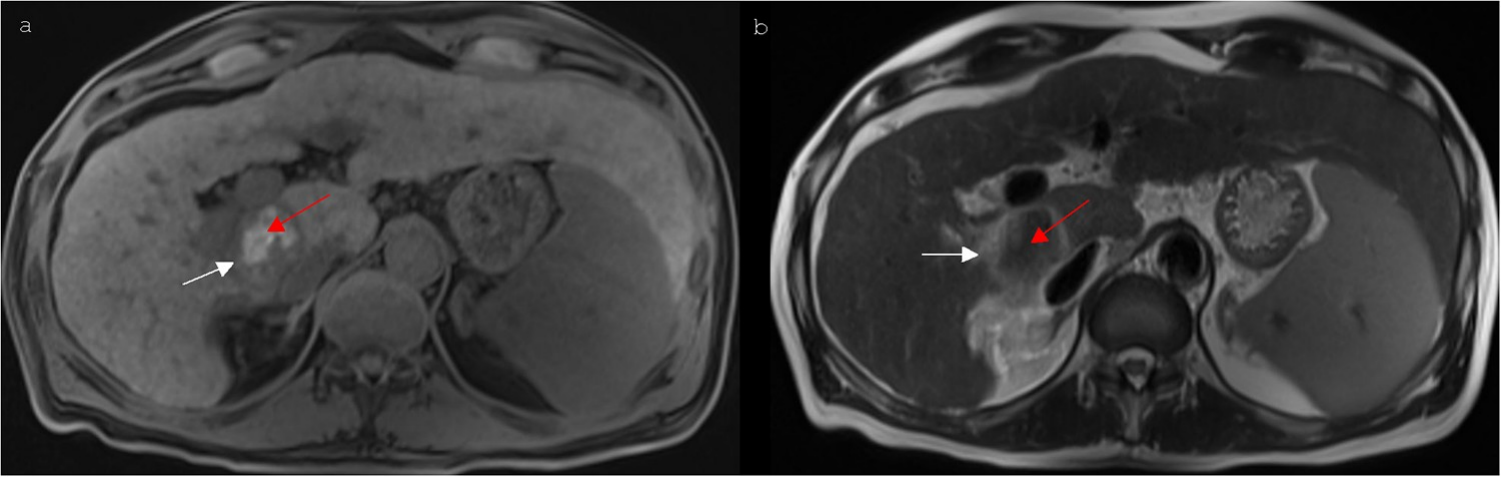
MR imaging appearance after IRE of a HCC located in segment IVb of a 75-year old man. (a) Postinterventional (1 day after IRE) T1 vibe3d fat suppressed contrast-enhanced MRI obtained in the portal venous phase shows an ablation area with a lot of hyperintense parts in its peripheral zone (red arrow) largely regressing up to the 6-weeks follow-up control (b).
